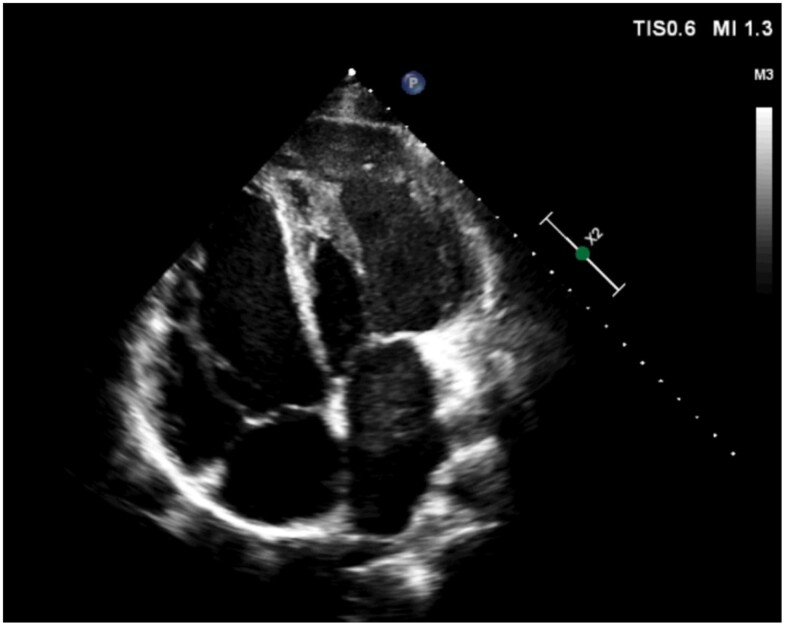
Transthoracic echocardiogram image. Four-chamber view in end-diastole showing biventricular dilatation with displacement of the interventricular septum towards the morphologically right ventricle in systemic position.
